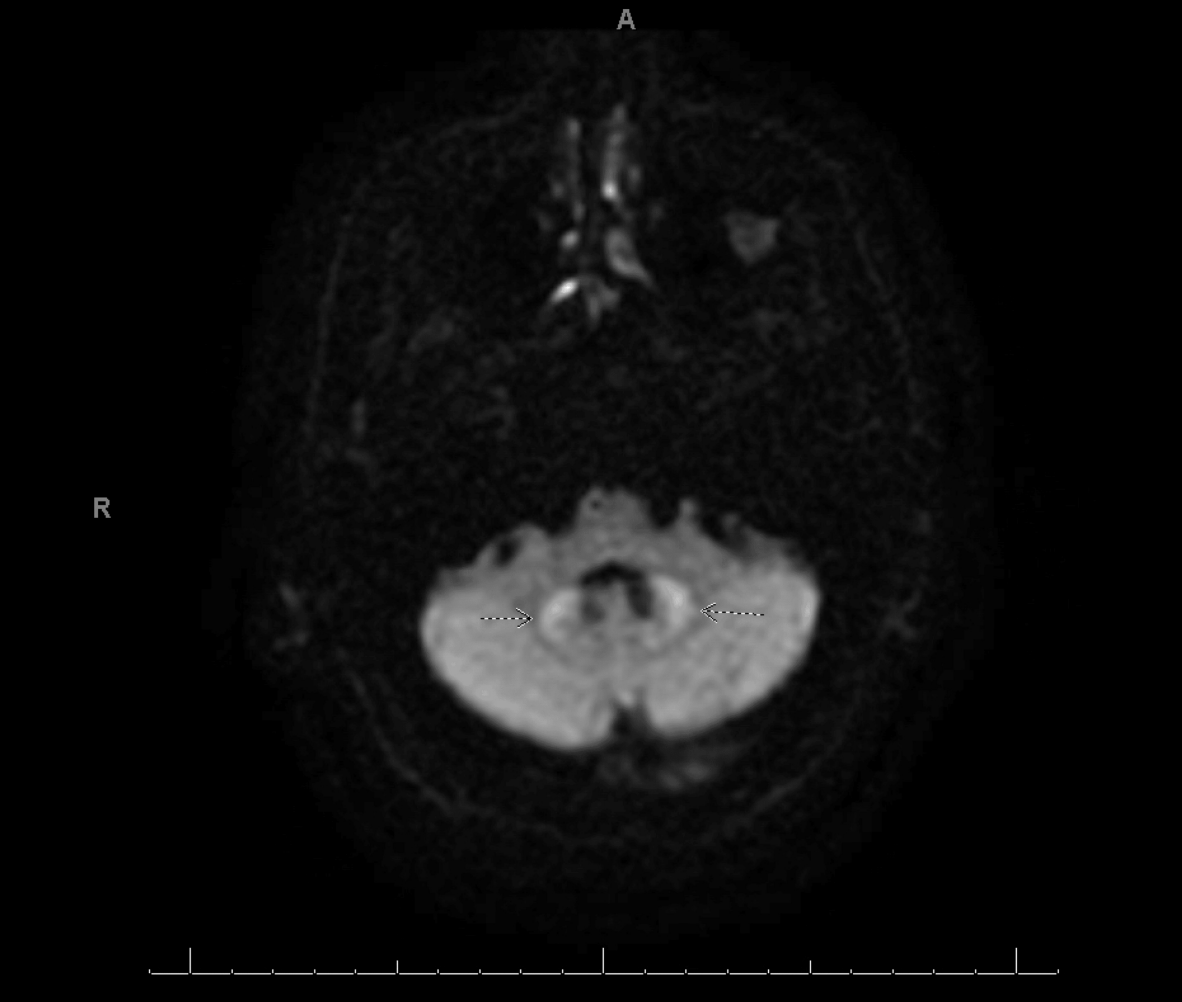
DWI Sequence Brain MRI. Brain MRI of this patient revealed T2 FLAIR bilateral hyperintensity in the dentate nuclei of the cerebellum (Figure 1) along with a bright signal on DWI (arrows on Figure 2).
